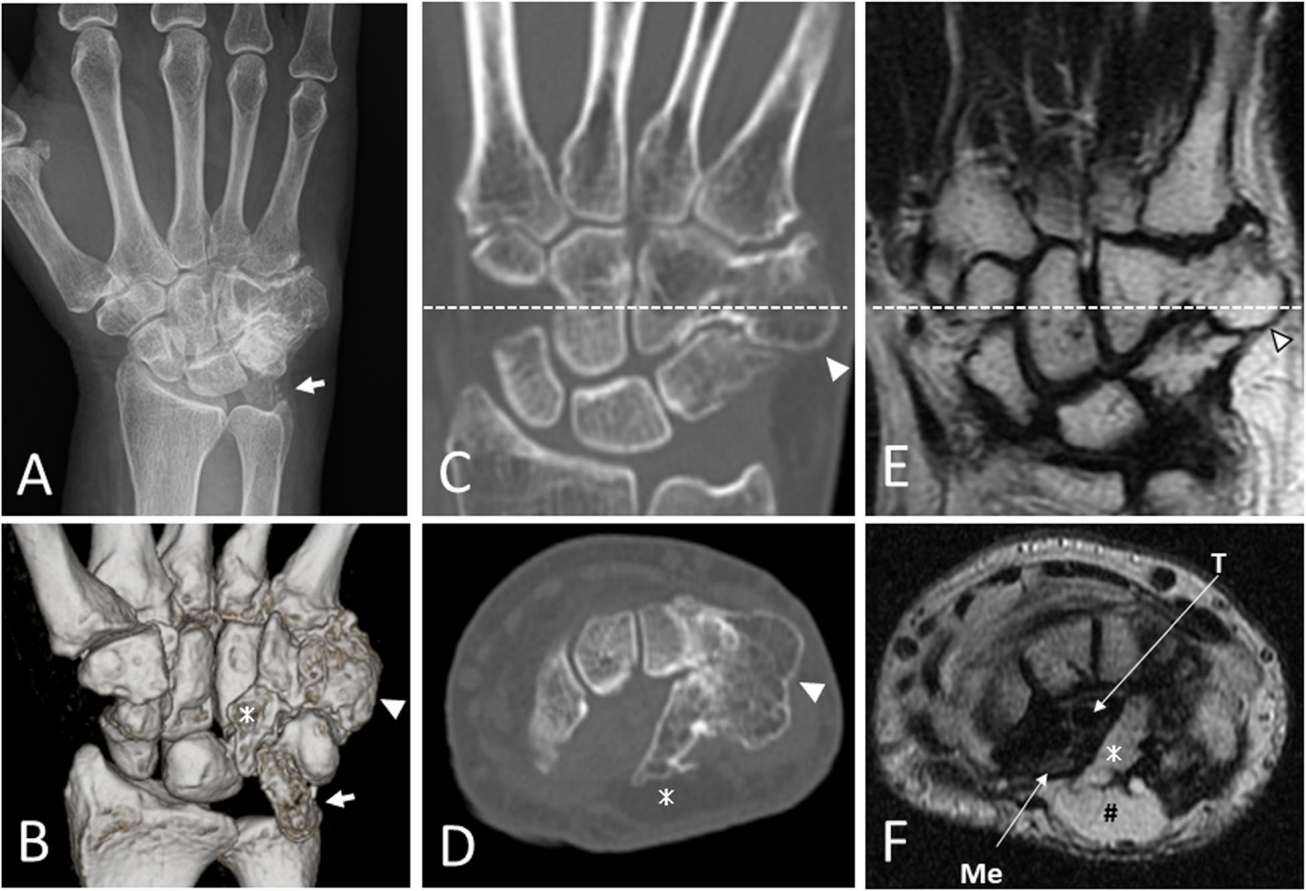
a Plain radiographs of the left hand at the first examination showed a morphological abnormality of the hamate and free body proximal to the pisiform (arrow). b–d Computed tomography showed a spiky bony protrusion (asterisk) into the carpal tunnel and rounded bony protrusion (arrowhead) on the dorsal–ulnar side of the hamate. e, f Magnetic resonance images showed that the bony protrusions were connected to the hamate body and had the same intensity as bone marrow. f Tenosynovitis of the flexor tendons (T) and edematous changes in the median nerve (Me) were confirmed. The subcutaneous lipoma (hash mark) with high intensity superficial to the spiky bony protrusion was found by chance
